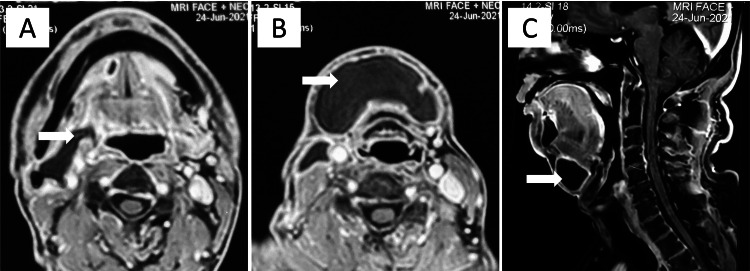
MRI with contrast of the face and neck showing (A) a non-enhancing thin-walled cystic lesion occupying the right submandibular region and continuing anteriorly into the sublingual space as the so-called “tail sign” (arrow); (B) the cystic lesion extending inferiorly into the submental space (arrow); and (C) submental cystic lesion (arrow) on sagittal section.
